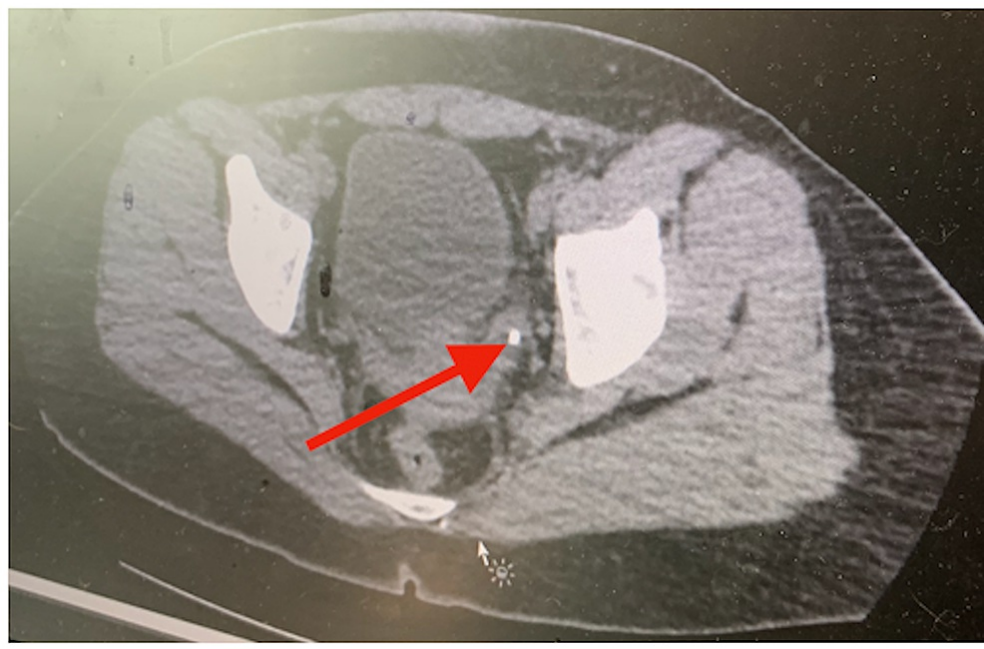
Non-enhanced axial CT scan images of the abdomen and pelvis demonstrate a calcific density at the left ureterovesical junction (red arrow).
